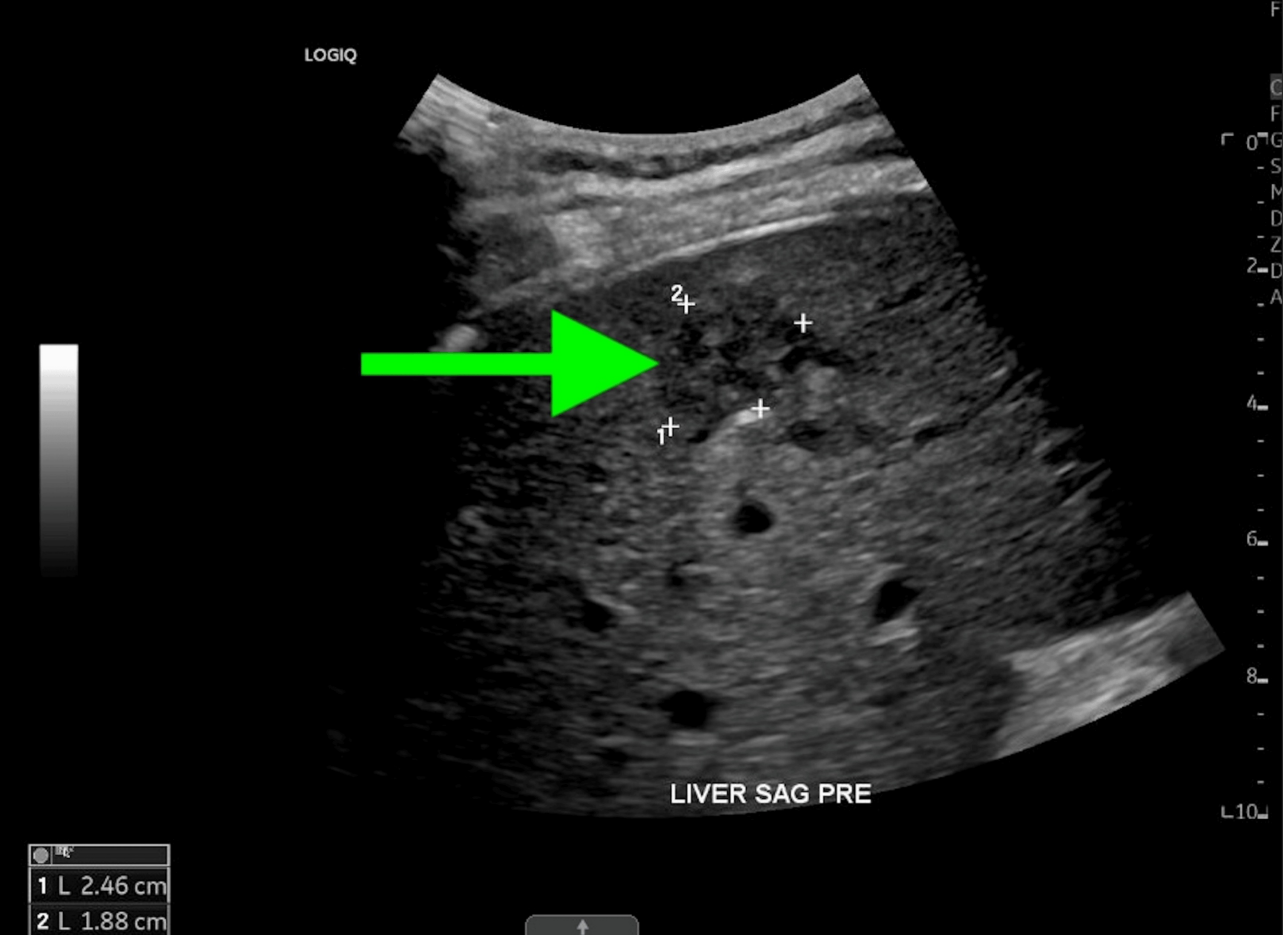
Ultrasound imaging of the liver The green arrow is demonstrating the hepatic mass during Interventional radiology core needle biopsy. Sonographic evaluation of the right hepatic lobe demonstrates a mixed echogenic mass.
